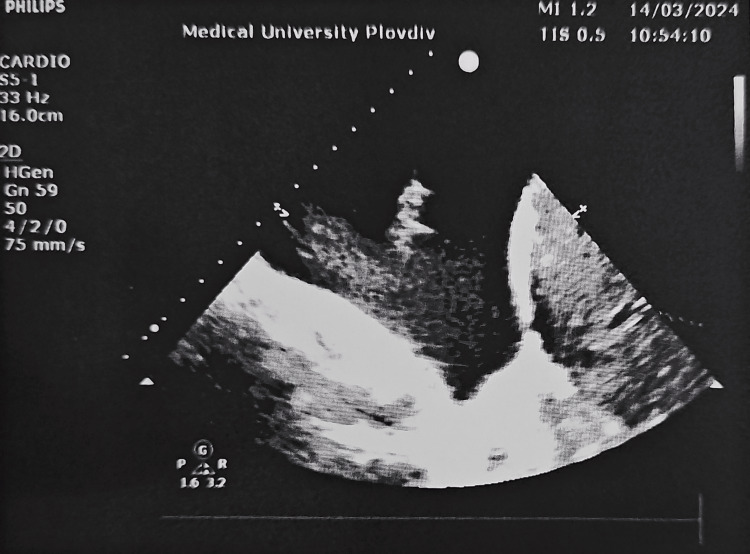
The ultrasound image of the smaller pleural effusion on the right side
